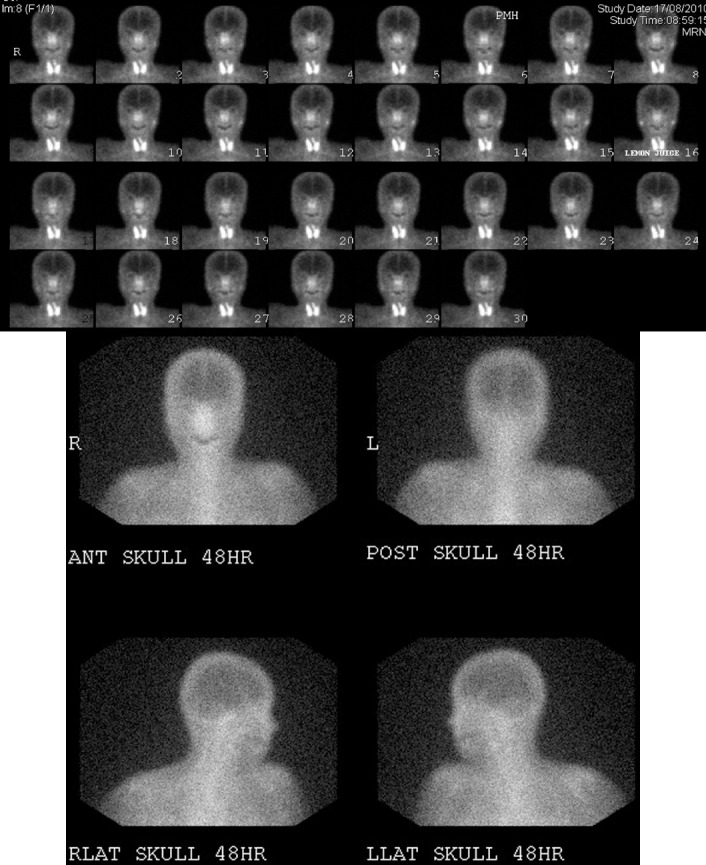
Images of a 57-year-old woman (Patient 7). (A) ^99m^Tc-pertechnetate scintigraphy shows marked decreased accumulation of tracer in submandibular glands and parotid glands. (B) Gallium scan of the bilateral parotid glands is normal
